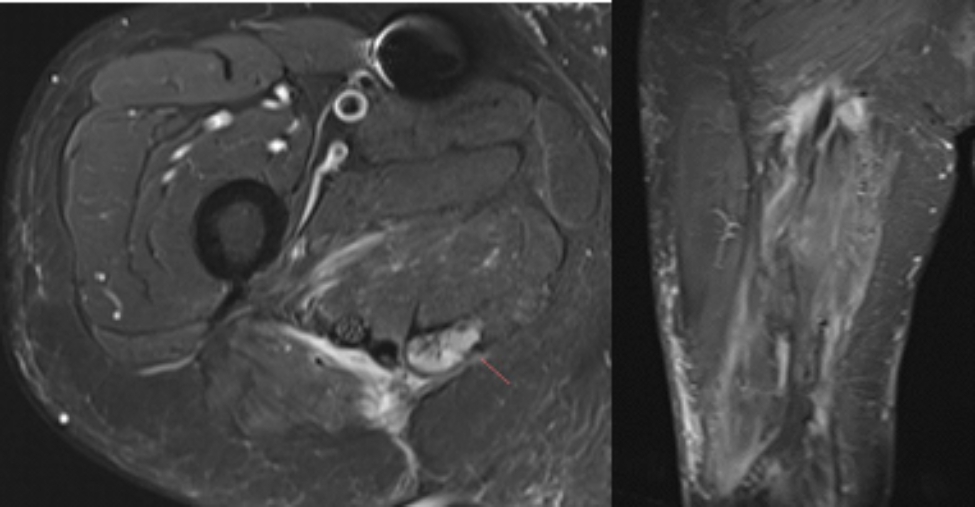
Post-recurrence preoperative T2-weighted MRI (left: axial; right: coronal
